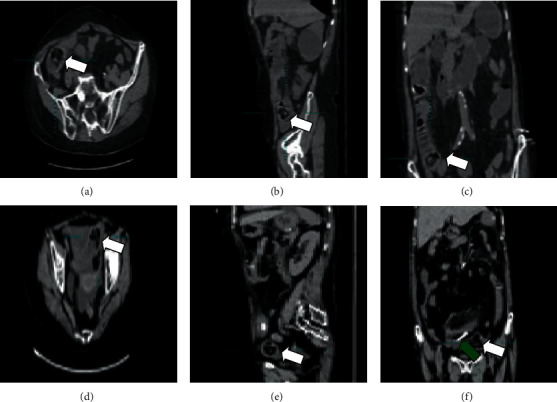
(a–c) Abdominal CT shows an intestinal mass considered to be a phytobezoar in the ileum (white arrow), proximal intestinal dilation, and intestinal effusion. (d–f) The green arrow indicates the transnasal ileus tube placed in the intestinal cavity on the 3rd day; the position of the phytobezoar (white arrow) is lower than previous position; small bowel obstruction is reduced.
